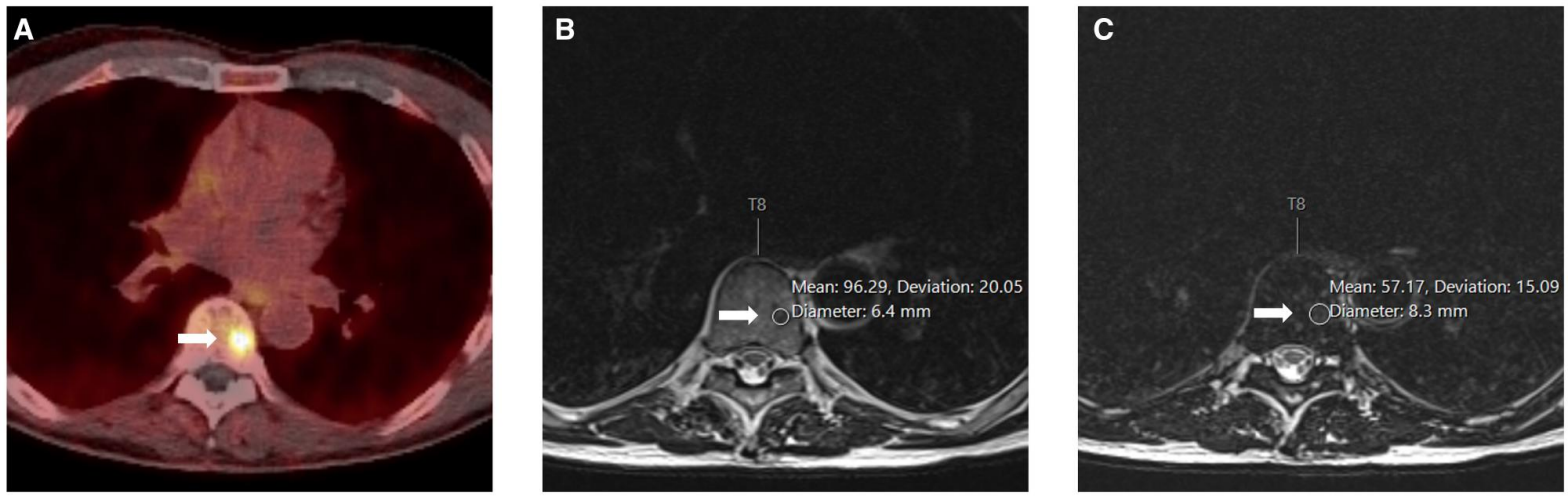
T8 vertebral body lesion (white arrow) in a 77-year-old male undergoing follow-up for previous prostate malignancy. (A) PMSA PET imaging demonstrates the focal avid lesion with SUV of 11.8 in axial formats (A). (B, C) Axial MRI Dixon sequence demonstrates greater than 40% drop-out within the lesion with mean signal intensities of 96.29 in-phase (B) vs 57.17 out-phase (C), suggestive of fat content.
